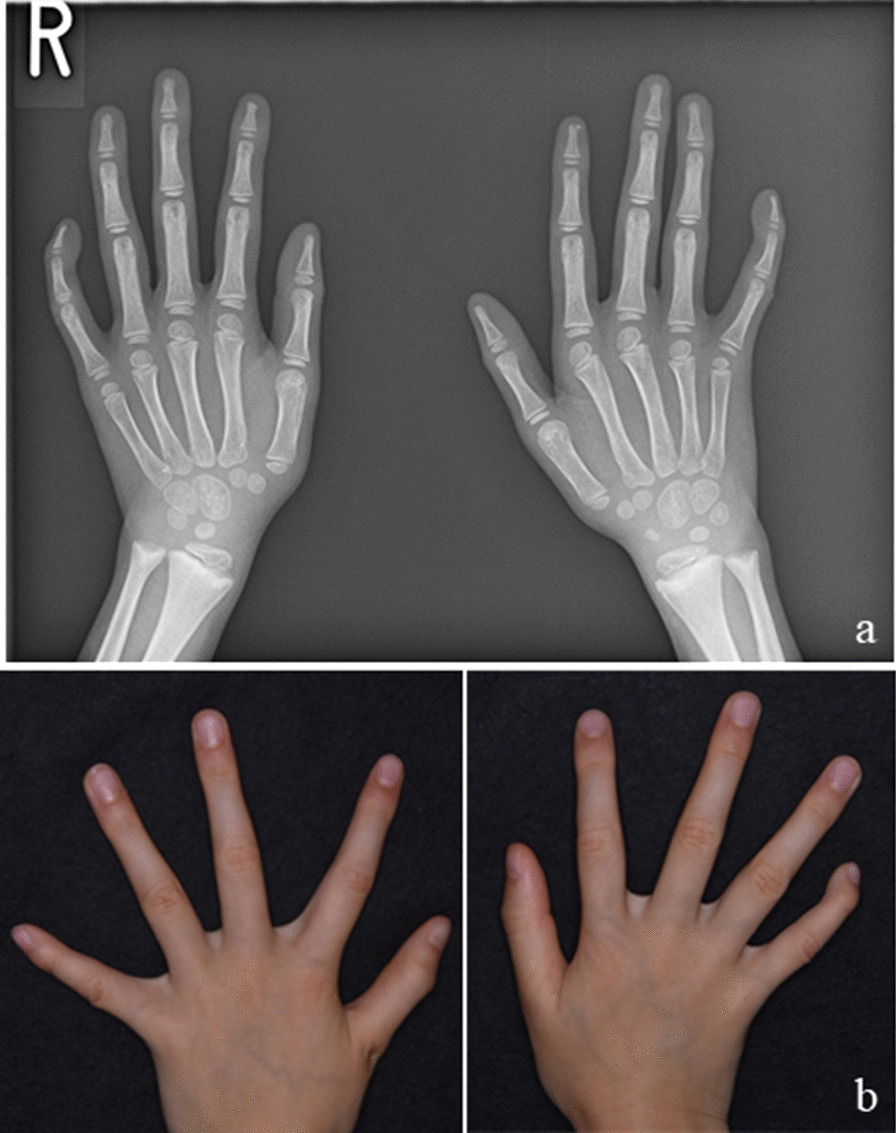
A hand-wrist radiograph and a photo of hands of the patient. a A hand–wrist radiograph was used to evaluate the skeletal age. b Cylindrically shaped fingers
